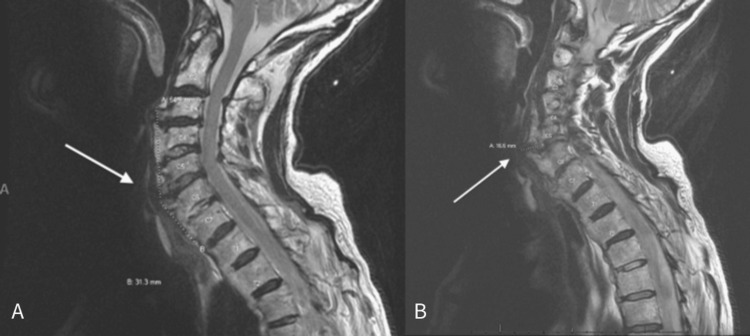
(A and B) Preoperative T2-weighted sagittal MRI of the spine demonstrating bulky, flowing osteophytes along the anterior aspect of the cervical and upper thoracic spine (white arrows). Original MRI image from Royal Hobart Hospital, Australia, 2025, reproduced with permission from Royal Hobart Hospital Radiology Department
